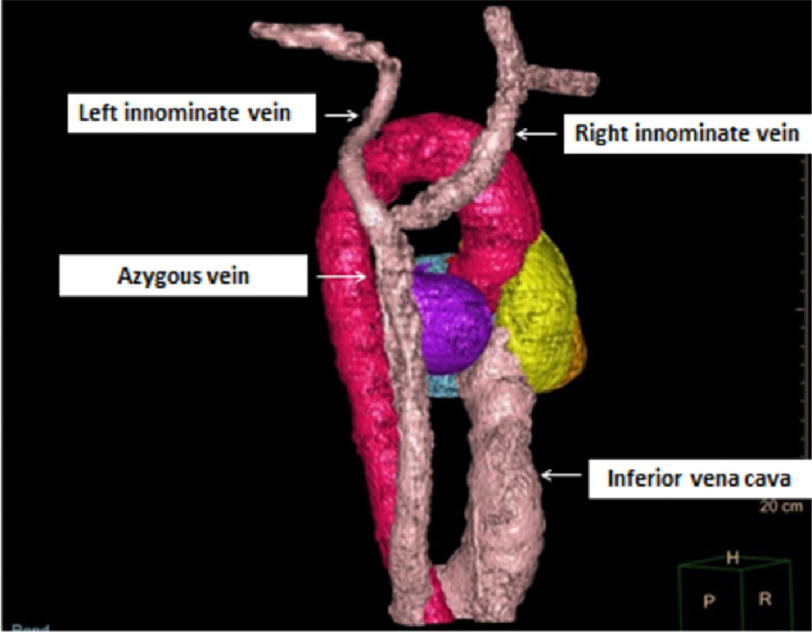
A CT chest with 3D reconstruction. It shows bilateral absence of the SVC with both innominate veins drain into the azygous vein that joins the IVC eventually. CT: Computerized tomography, SVC: superior vena cava, IVC: inferior vena cava.
